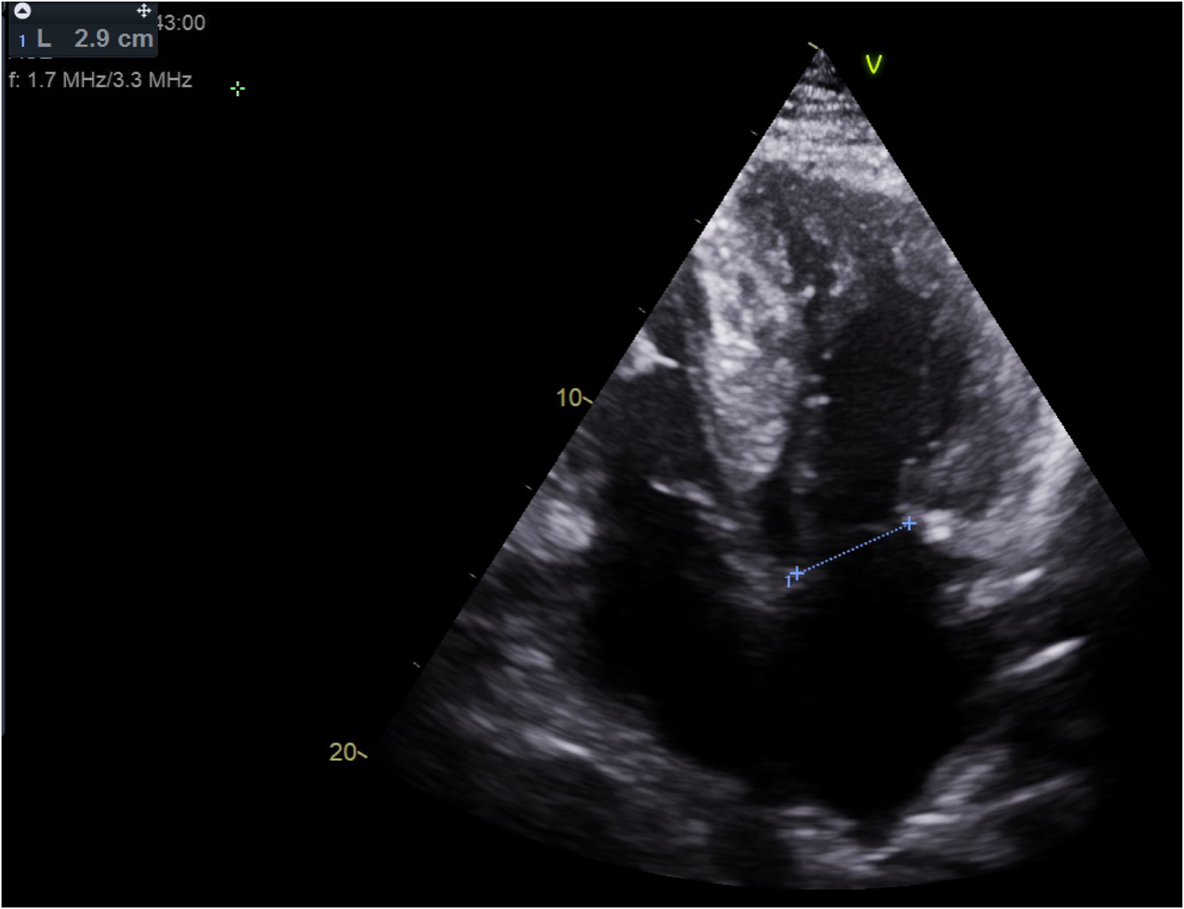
Anterior-posterior diameter of mitral annulus, apical four chamber view (4CH)
